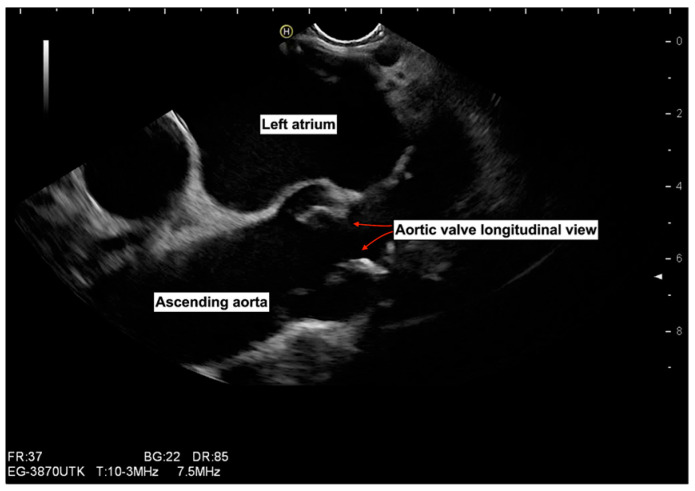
Endoscopic ultrasound demonstrated the morphology of left atrium in another view and the relation to longitudinal view of aortic valve and ascending aorta.
